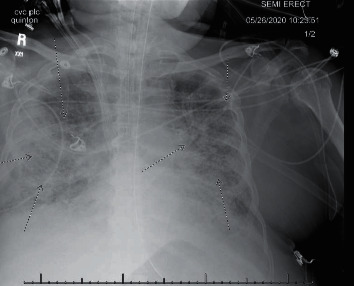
Portable chest X-ray with lungs demonstrating extensive diffuse infiltrates with worsening aeration from the prior chest X-ray.
